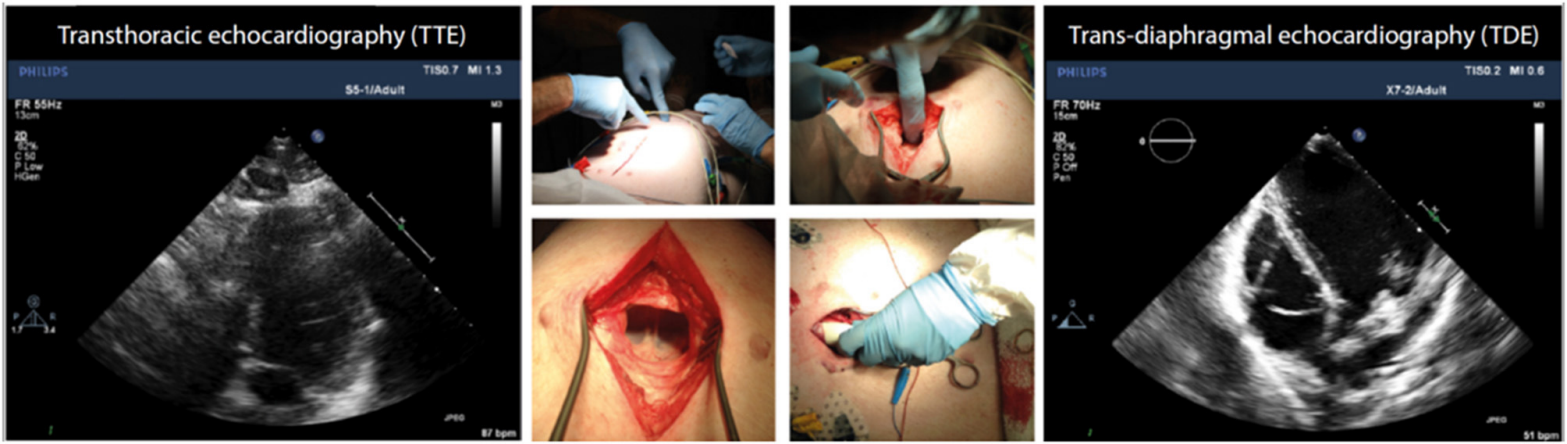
Trans-thoracic and trans-diaphragmal echocardiographic approach (12).
